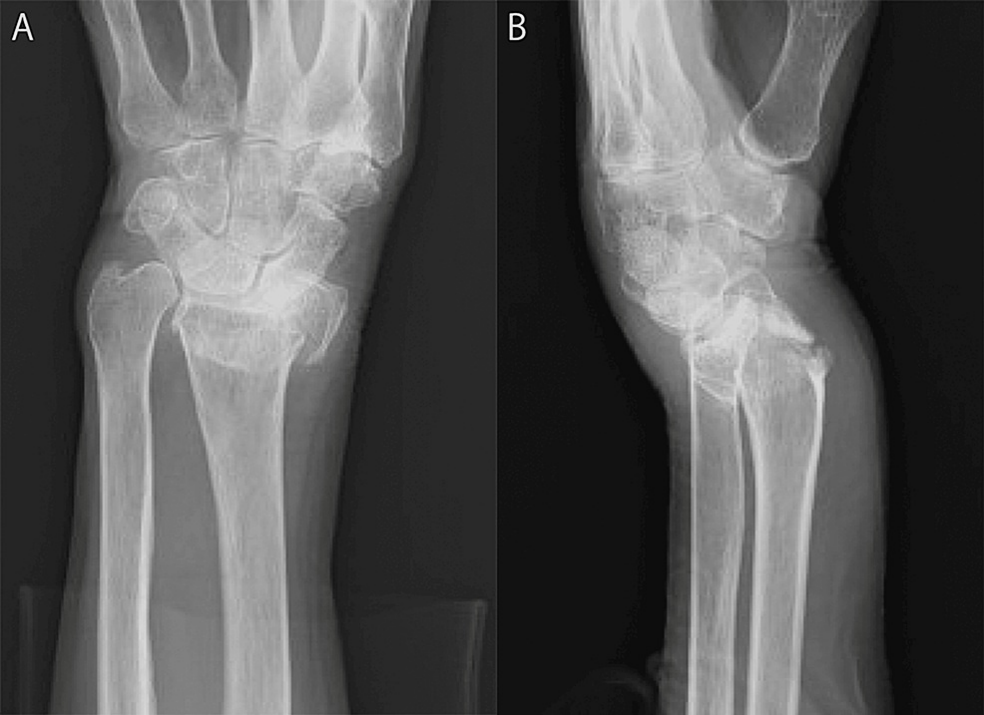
Distal radius fracture with malunion of the volar cortex of the distal radius. Frontal view (A) and lateral view (B) on plain radiography.
